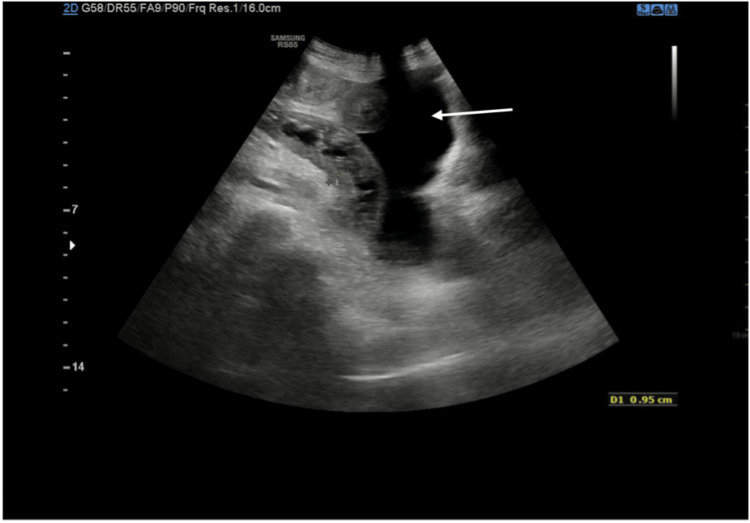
Follow-up abdominal ultrasound showing increased ascites and abdominal wall thickening
